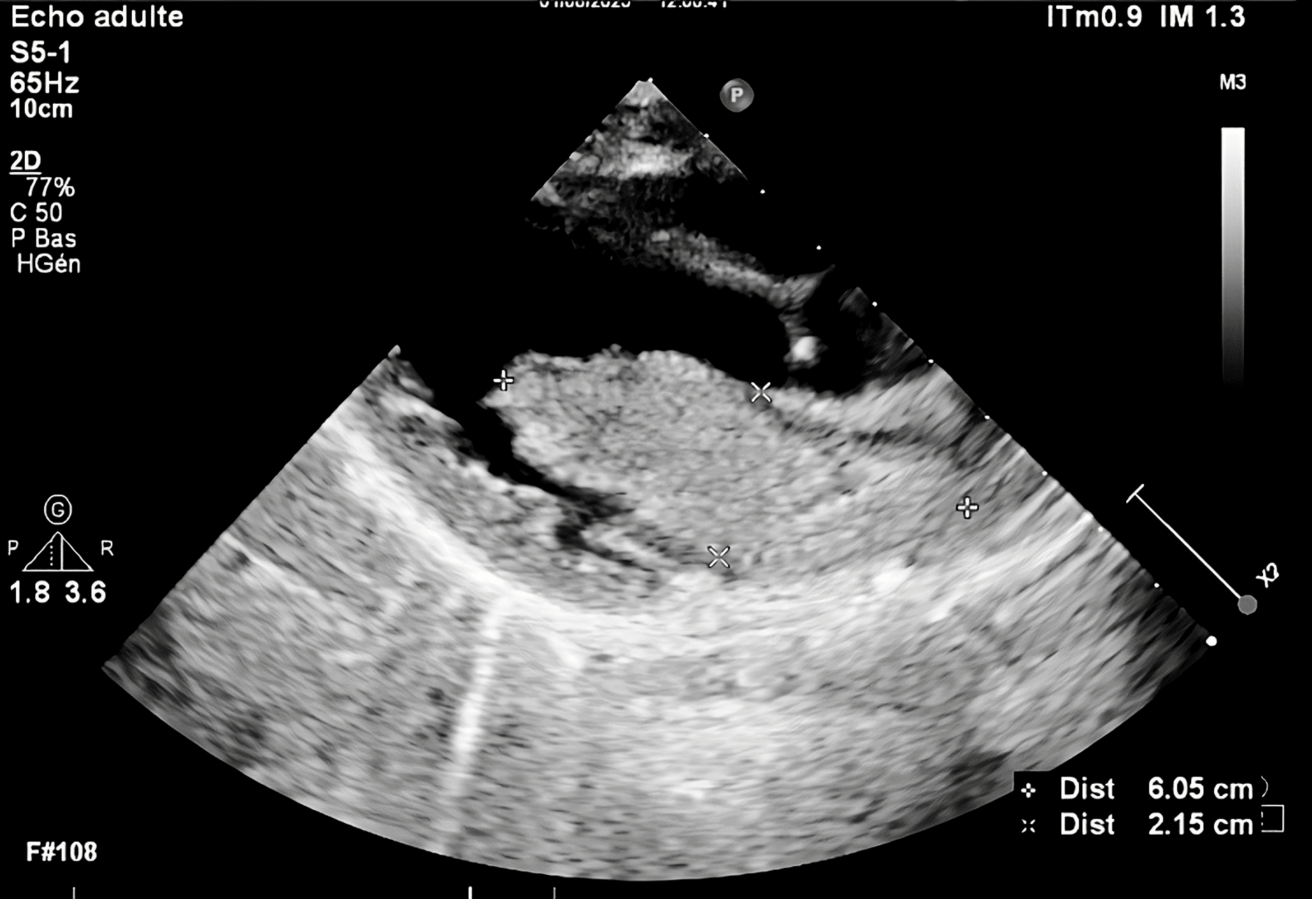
Transthoracic echocardiography showing a large, homogeneous, mobile mass occupying the left atrium, consistent with atrial myxoma
